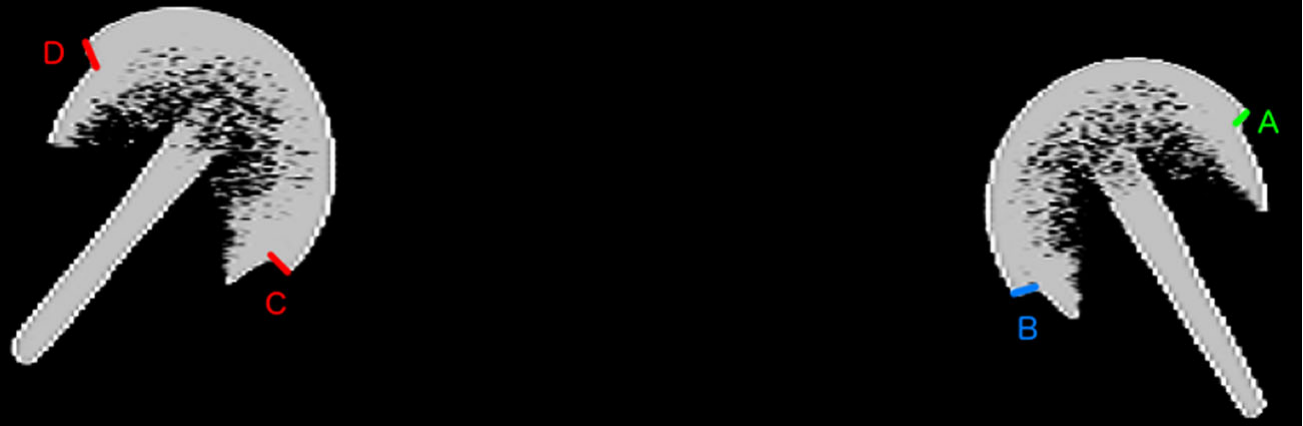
A 2D CT coronal slice of the patient with the mismatched left hip resurfacing (*right*) and the patient’s previous right hip resurfacing (*left*) in view. The femoral head of the mismatched component is not sitting centrally in the acetabular component with* B* being 1.5 times greater than* A*. On the previous right hip resurfacing, with correctly paired components, the femoral head is centrally placed (*C* to* D*)
